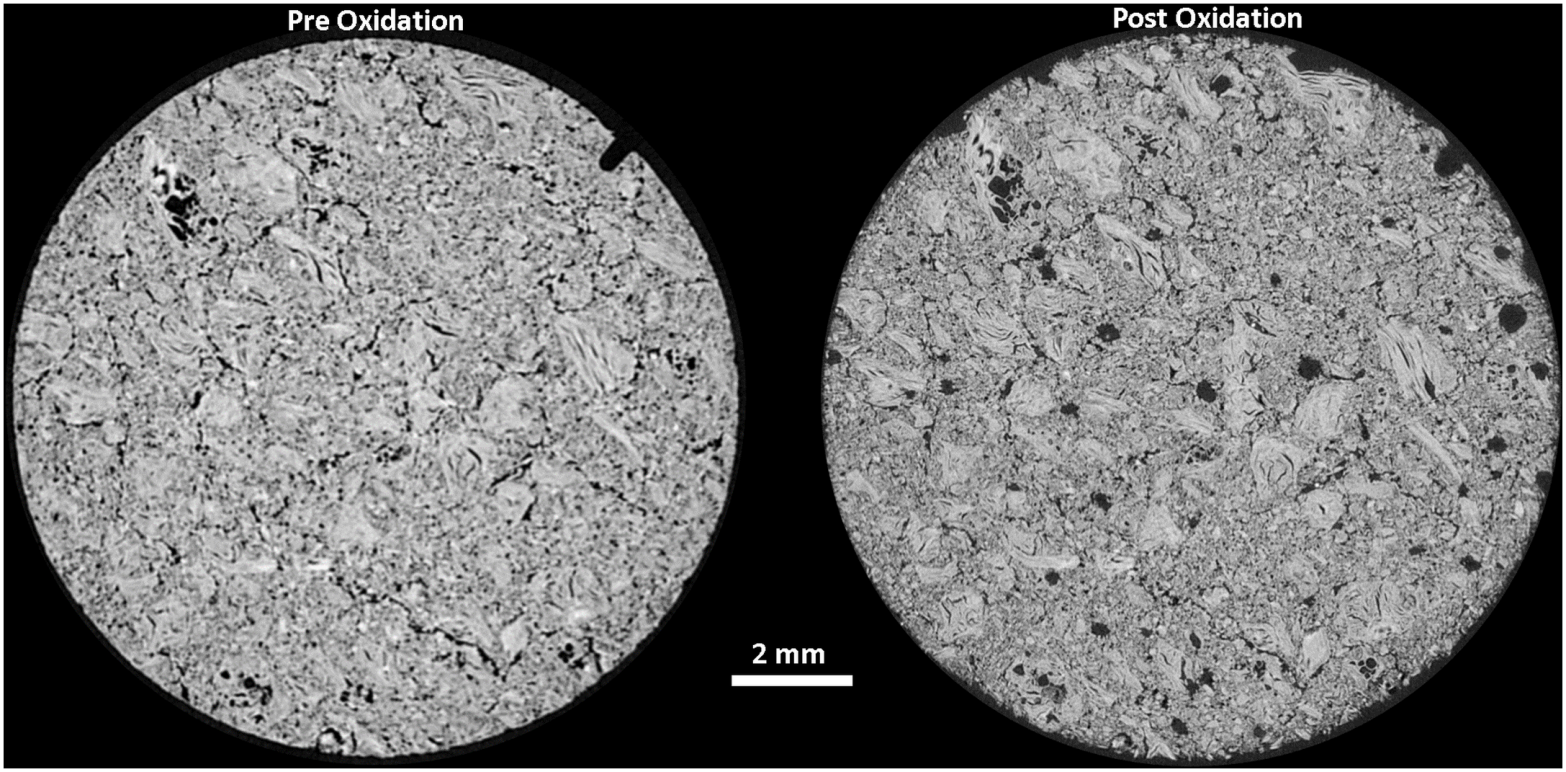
X-ray tomograms from PGA graphite pre and post oxidation at 650°C in air.
